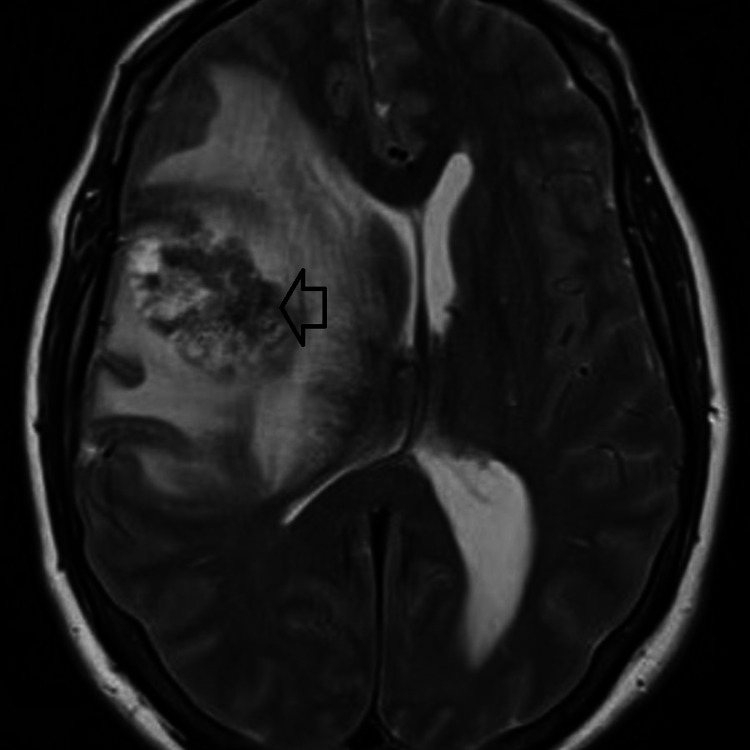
MRI of brain with and without contrast showing right lateral frontal intra-axial heterogenous enhancing mass measuring 4.4 x 4.0 x 3.7 cm with surrounding edema and right to left midline shift
